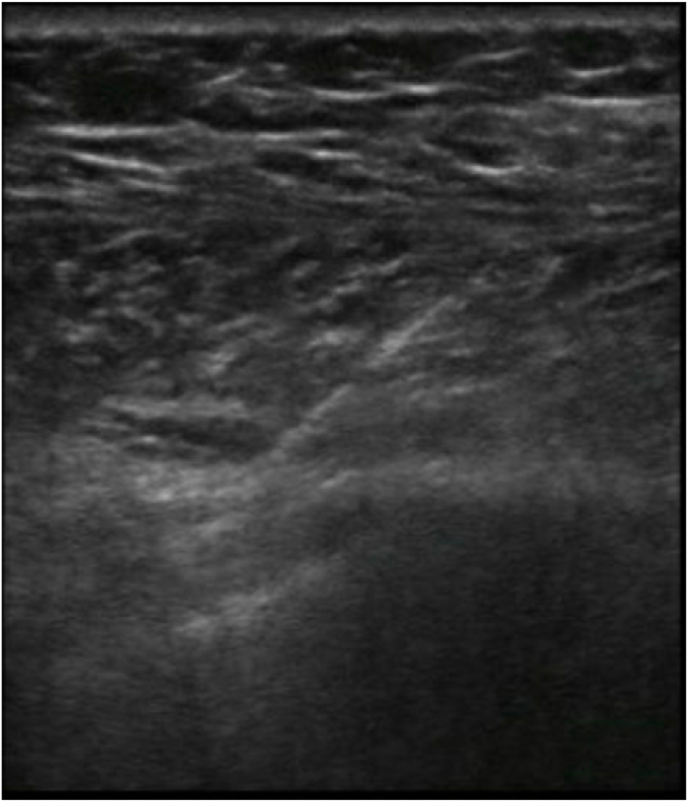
Ultrasound of lead placement.
